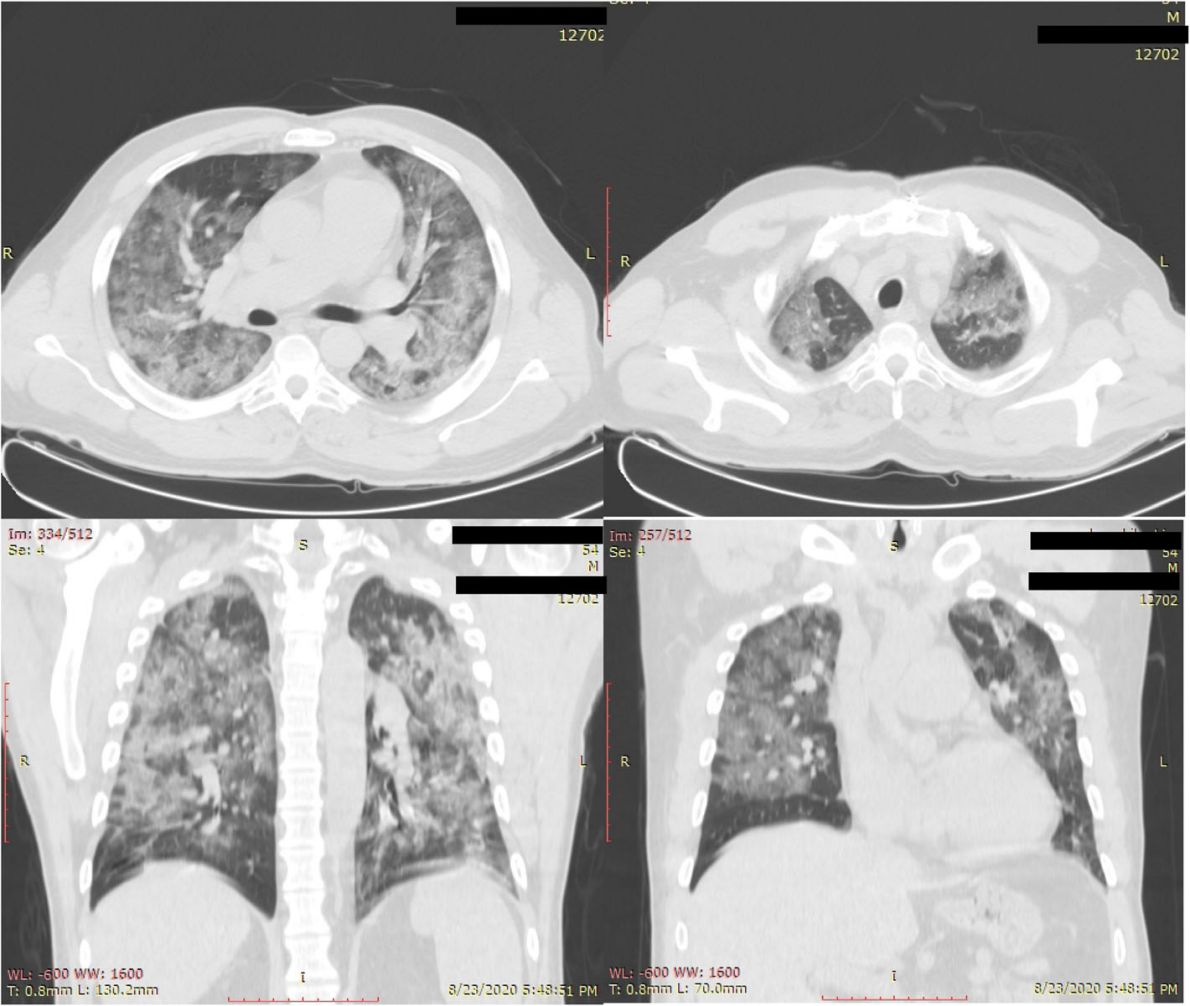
Sixty-four-year-old male presented with cough. His PCR test was positive. CT chest is showing diffuse ground glass opacities with consolidative changes. There is associated vascular enlargement within the GG areas. Lung involvement is about 80%, i.e., CT severity score is 5
